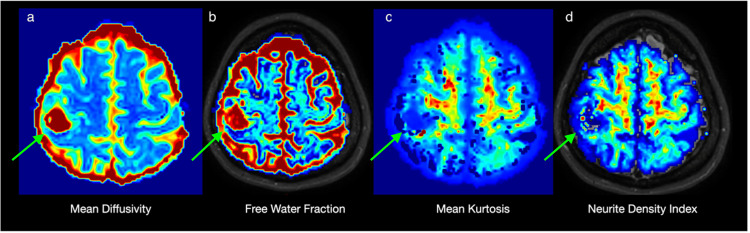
MRI advanced diffusion techniques When the lesion was evaluated with advanced diffusion techniques, an increase in MD (a) and a decrease in MK (c) were observed. An increase in FWF (b) was noted in and around the lesion. No increase in NDI (d) indicative of the presence of axons or dendrites was seen within the lesion. MD: Mean diffusivity; MK: mean kurtosis; FWF: free water fraction; NDI: neurite density index.
